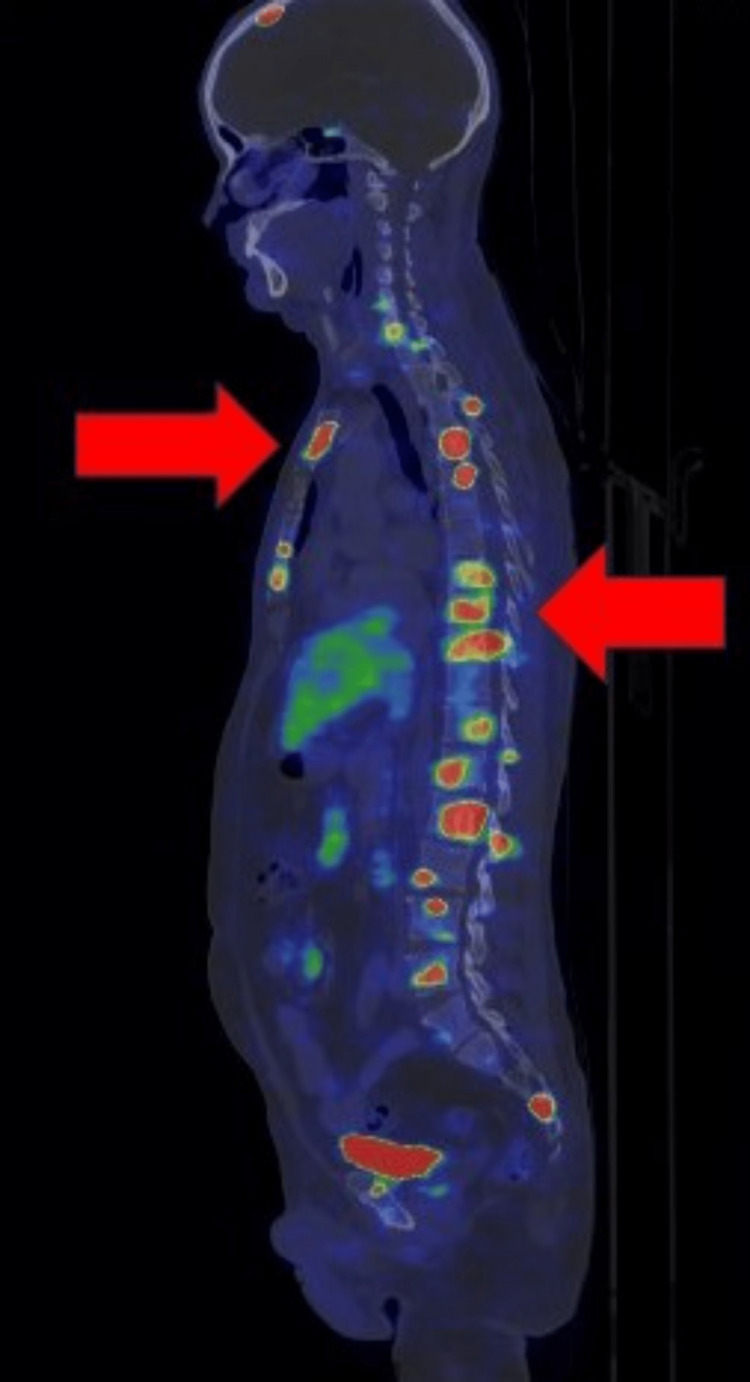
Sagittal 68Ga-DOTANOC PET-CT image showing tracer avid extensive skeletal metastases in the sternum (red arrow on the left) and multiple vertebrae (red arrow on the right).
